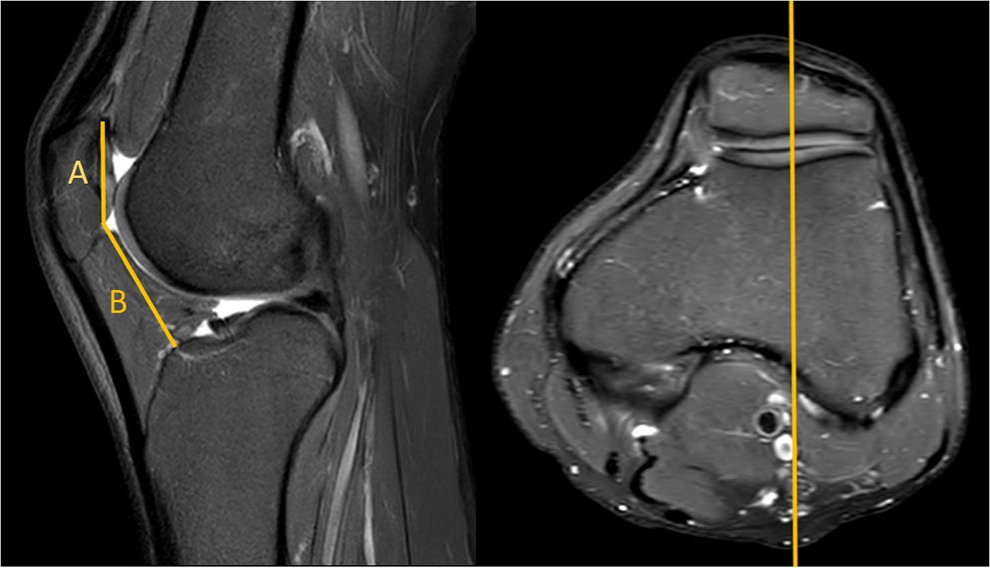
MRI true sagittal view. Caton–Deschamps (CD) index measurement with one slice. B distance from the patella to the tibia and A patellar cartilage length. CD: B/A
